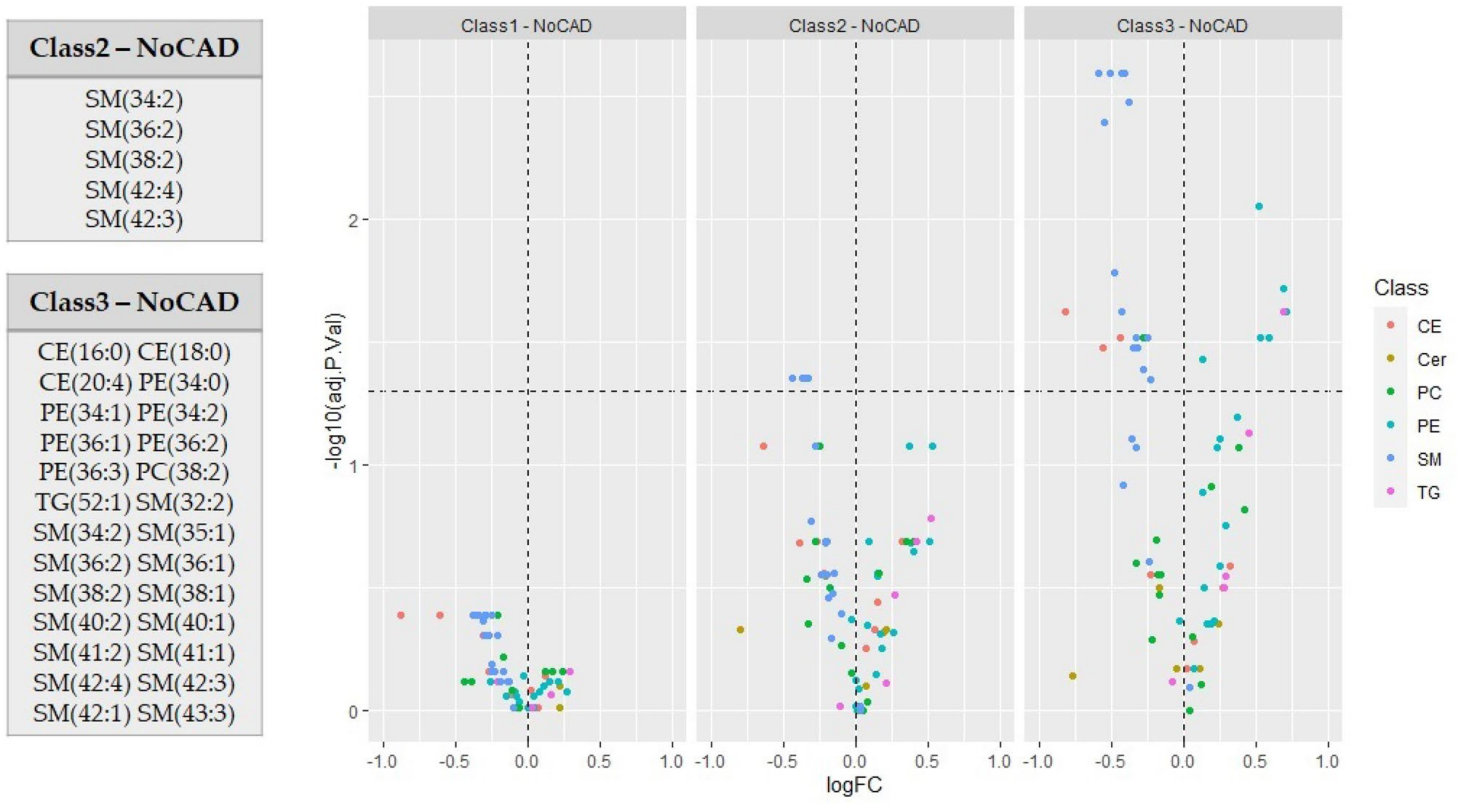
Lipid profile analysis based on CTA score annotations. Volcano plots showing the differentially expressed lipids (adj. p-value threshold = 0.05) among CTA score annotation Classes relative to No CAD subjects. Lipids are colored according to lipid class (CE, Cer, PC, PE, SM, TG). The panels on the left report in details lipids that are significantly dysregulated in comparison Class 2 vs No CAD and Class 3 vs No CAD.
